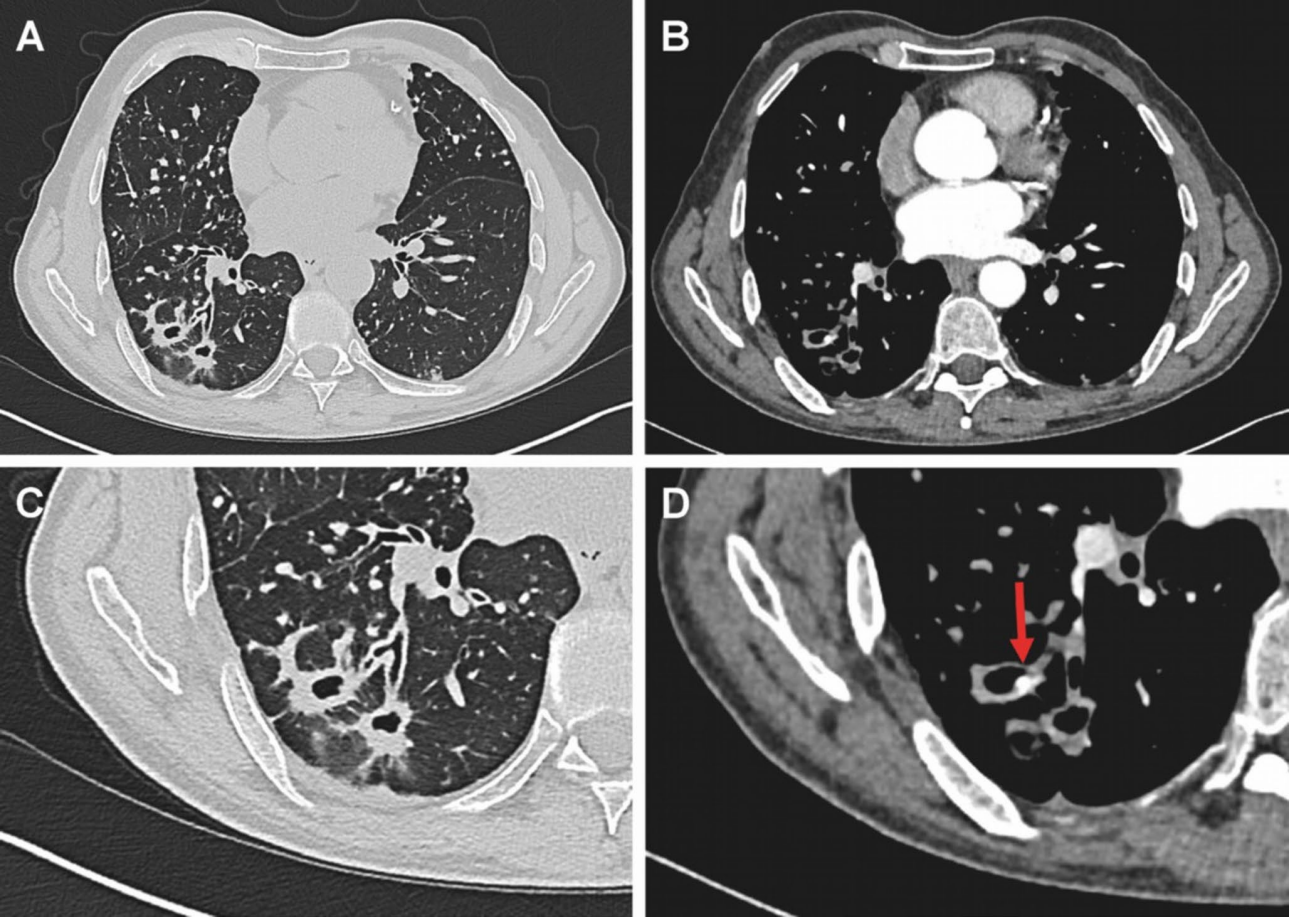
CT imaging of a patient with bipulmonary tuberculosis and cavitary pulmonary disease. Axial non-contrast (A) and contrast-enhanced CT (B) showing bilateral miliary nodules and multiple cavitary lesions in the right lower lobe with progressive consolidation of the cavities. C Magnified axial view highlighting the partially consolidated cavitary lesions in the right lower lobe. D Contrast-enhanced axial CT with detection of a pseudoaneurysm (red arrow) within a cavity in the right lower lobe, originating from a subsegmental branch of the pulmonary artery — indicating a potential source of hemoptysis.
